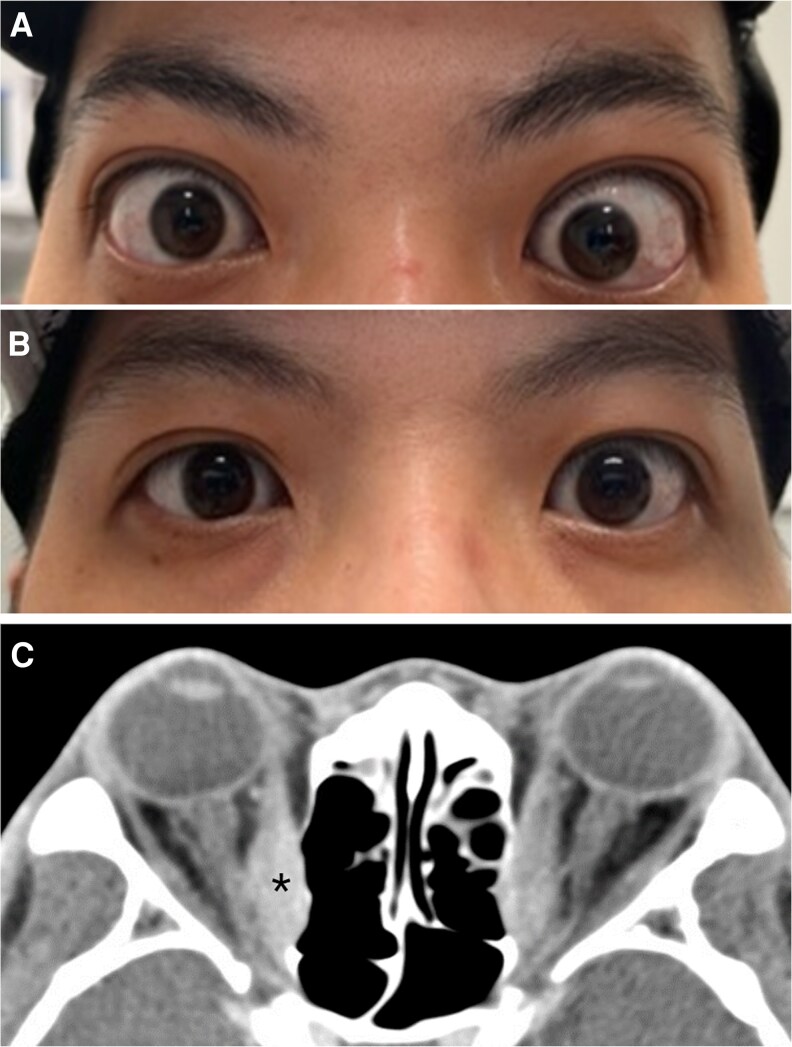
Graves’ ophthalmopathy with bilateral asymmetric proptosis with lid retraction (A) improved after teprotumumab (B). Computed tomography orbit showed bilateral proptosis, enlargement of rectus muscle (*), and crowding at the level of the orbital apex (C).
